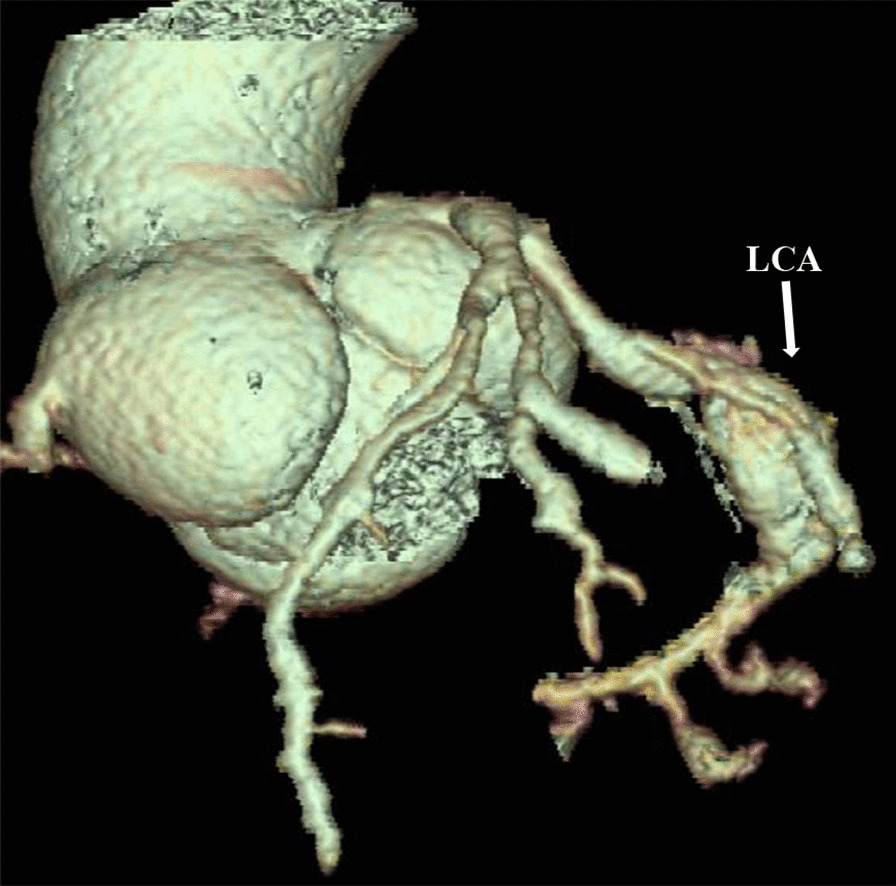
Three-dimensional CT reconstruction of the aorta showing dilation of the right coronary artery and aneurysm of the left coronary artery (Patient 10)
